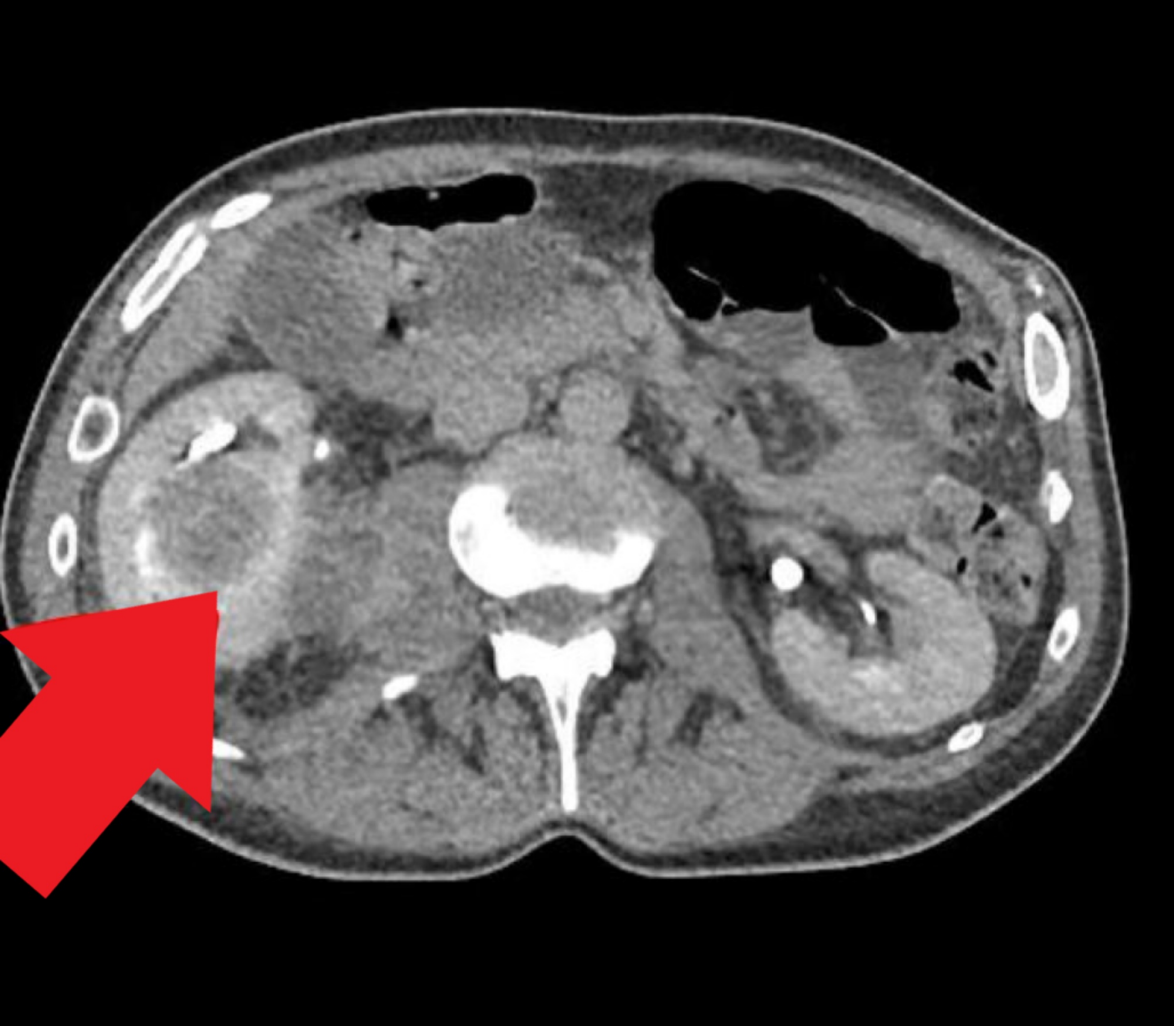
Right renal abscess on the computed tomography scan
